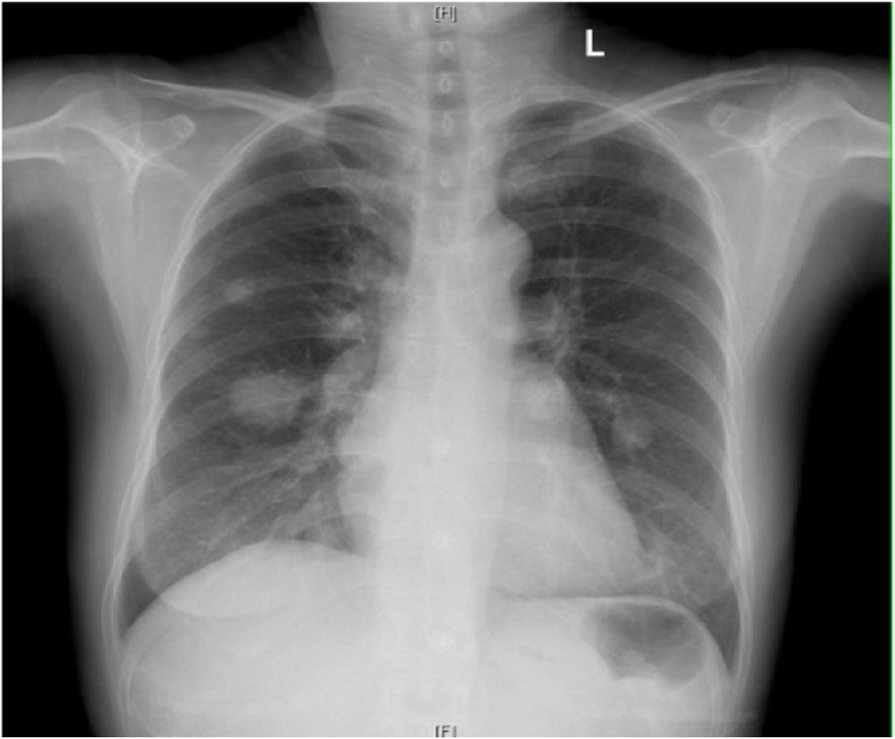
After the second admission, the preoperative chest radiograph showed nodular space in the right lung
